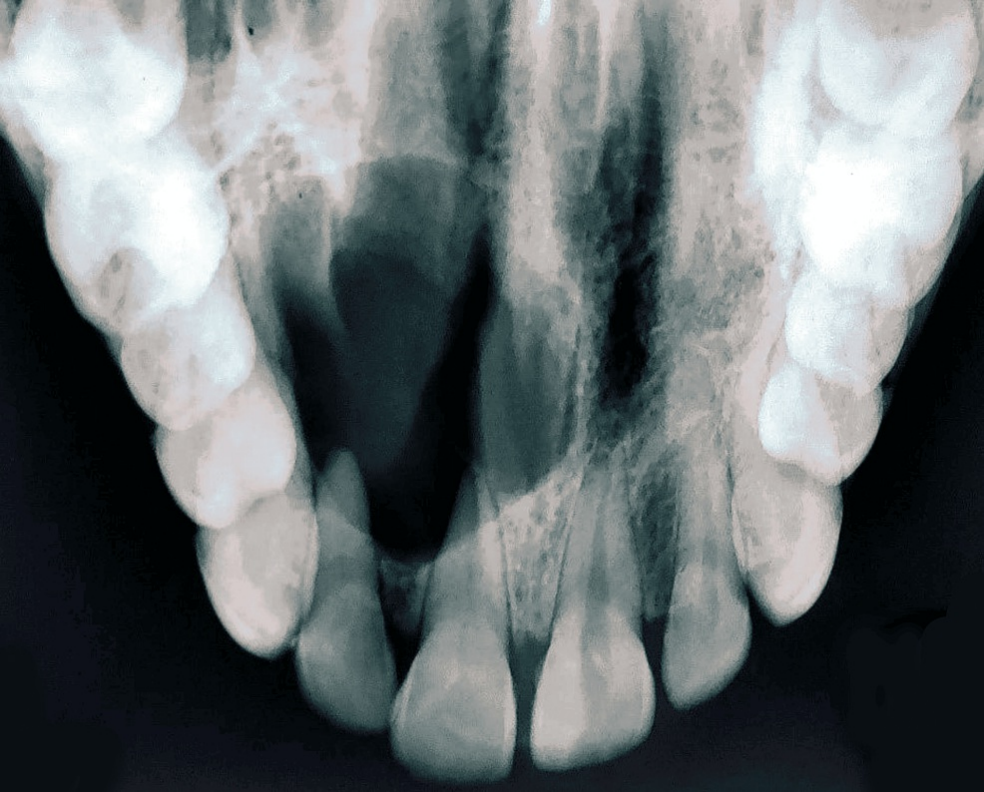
Occlusal radiograph showing the lesion in 11-14 region. Image Credit: Prasanna Sonar.
